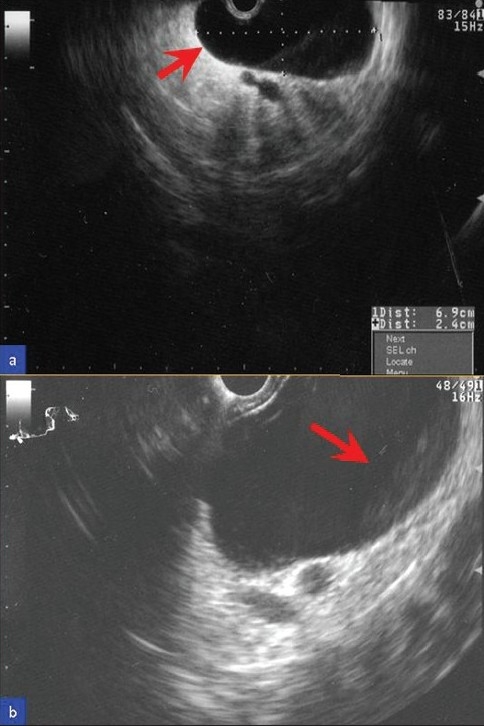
Ultrasound image of the pancreatic cystic lesion. (a) EUS showed a 6.9 × 2.4 cm cyst (arrow) in the pancreatic body–tail region without septae or solid component. (b) Under higher magnification, there is a subtle layering of debris (arrow) within the cyst
